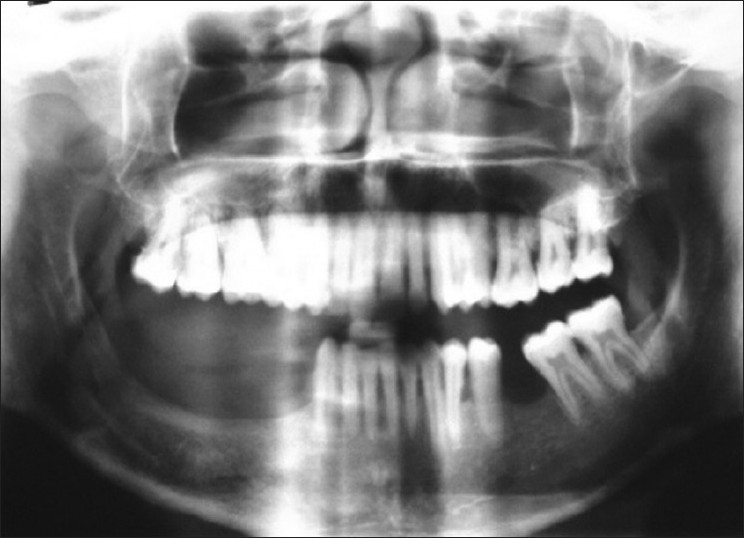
Postoperative panoramic radiograph, 1 year after curettage, showing no recurrence
